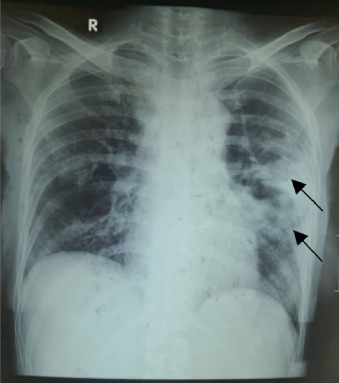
Thoracic radiograph showing left paracardial reticulogranular infiltrates, indicating pneumonia.
